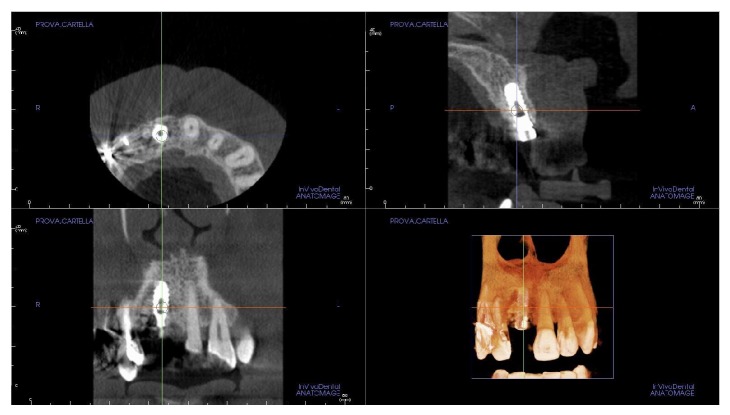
A second small FOV CBCT with 3D reconstruction confirmed optimal implant placement in the regenerated bone.
